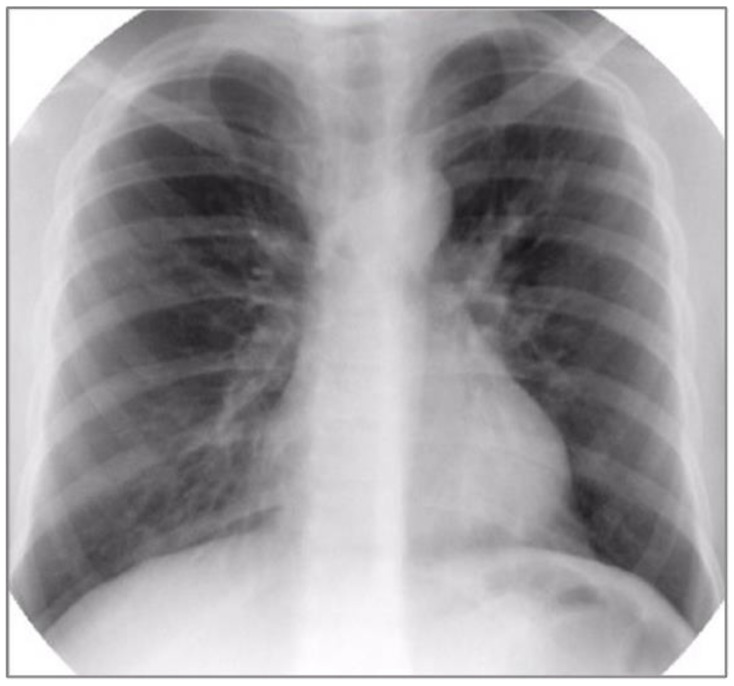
Chest radiography showing diffuse interstitial infiltrates, predominantly perihilar.
